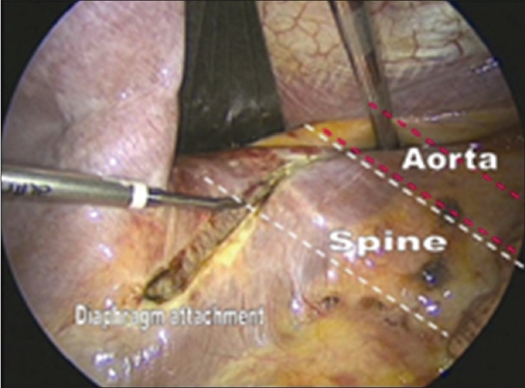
Partial detachment of the diaphragm using an ultrasound knife
